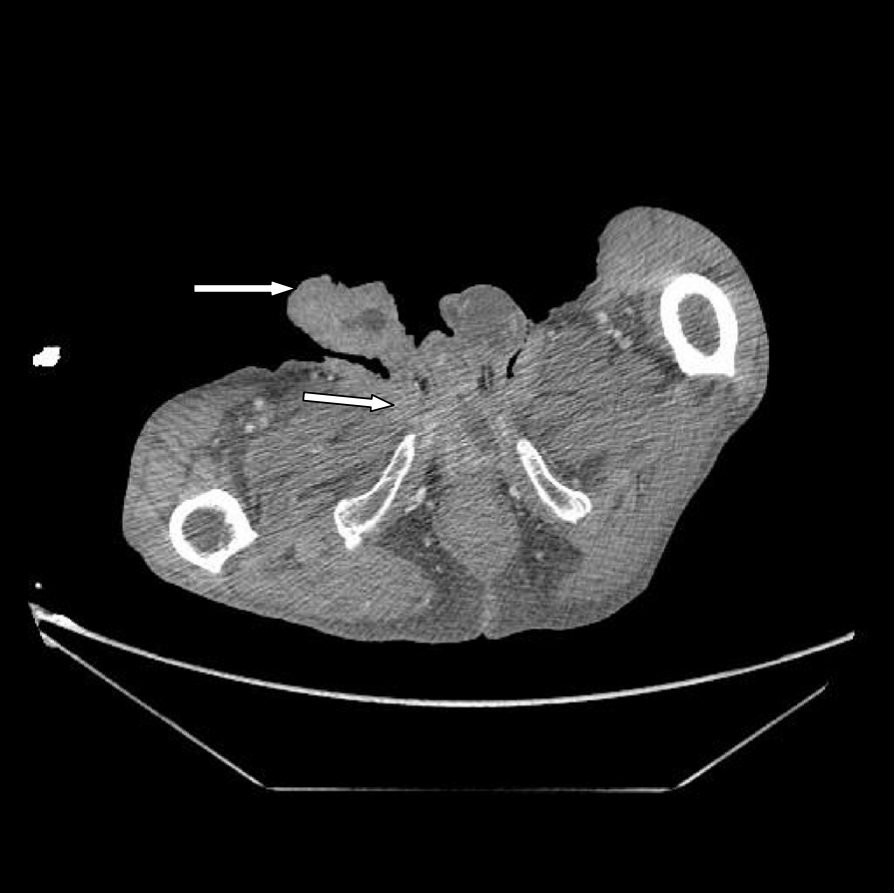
Computed tomography of the perineum showing the tumor spreading into the perineum and base of the scrotum (white arrows).
